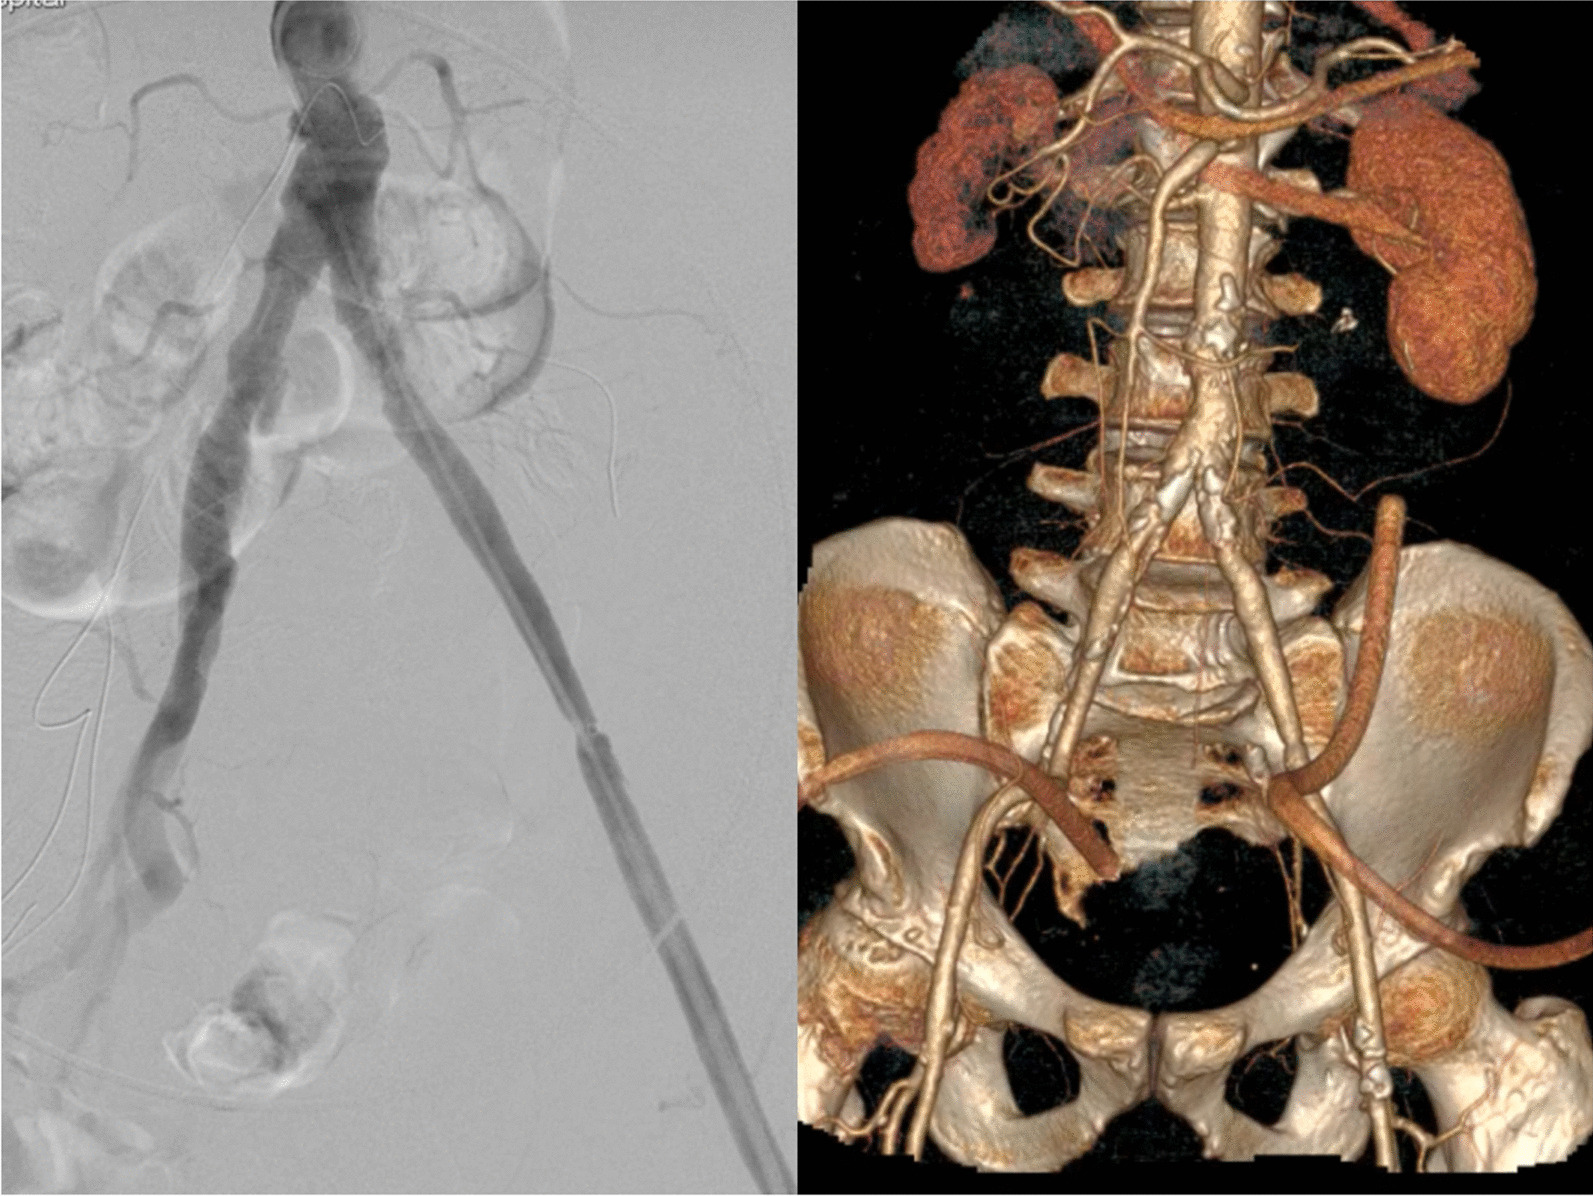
Intraoperative angiography and 1-month CT scan showing patency of the graft in absence of stenosis or dilatation
